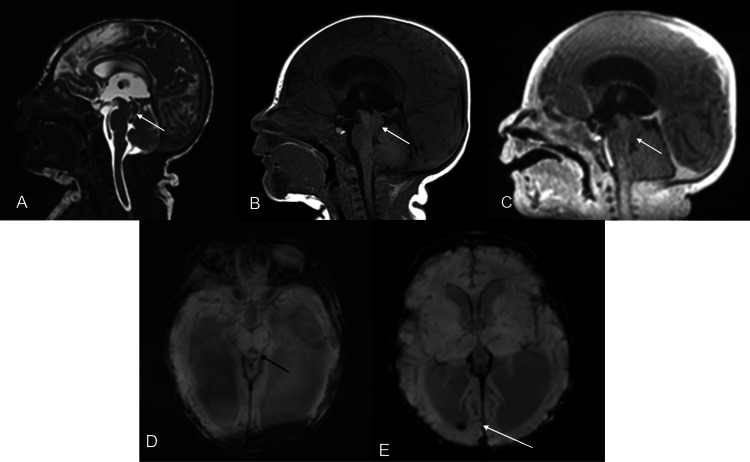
Sagittal T2 CISS (A), sagittal T1W pre (B) and postcontrast (C) MR images of the brain demonstrating a nonenhancing isointense T1/T2 lesion noted in the aqueduct, extending into the upper fourth ventricle (short white arrows). No restricted diffusion was noted (not shown). There is susceptibility noted within the lesion (black arrow) as well as a small amount of right intraventricular hemorrhage (long white arrow) on the SWI images (D, E) within the dilated ventricles. CISS: Constructive interference in steady state; MR: magnetic resonance
